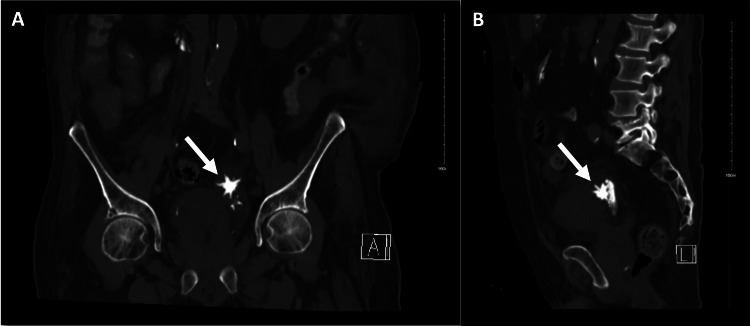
(A) coronal and (b) sagittal views of non-contrast CT abdomen showing spiculated Jackstone calculus (arrow) inside a bladder diverticulum
